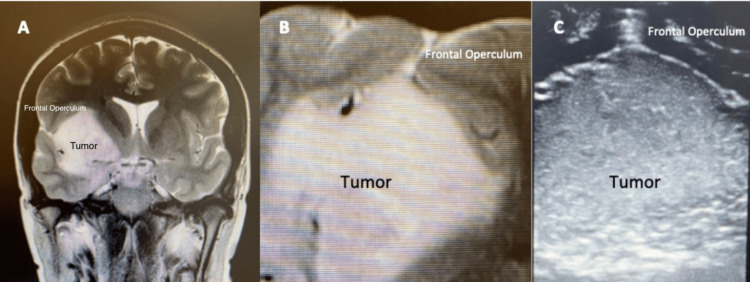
iUS images correlate with the preoperative MR images (A) Preoperative T2 coronal image depicting an insular LGG. (B) Direct correlation between the preoperative MR image and iUS image obtained during tumor localization. The hyperintense zone correlates with the hyperechoic zone in the iUS image. iUS: intraoperative ultrasound; LGG: low-grade glioma
